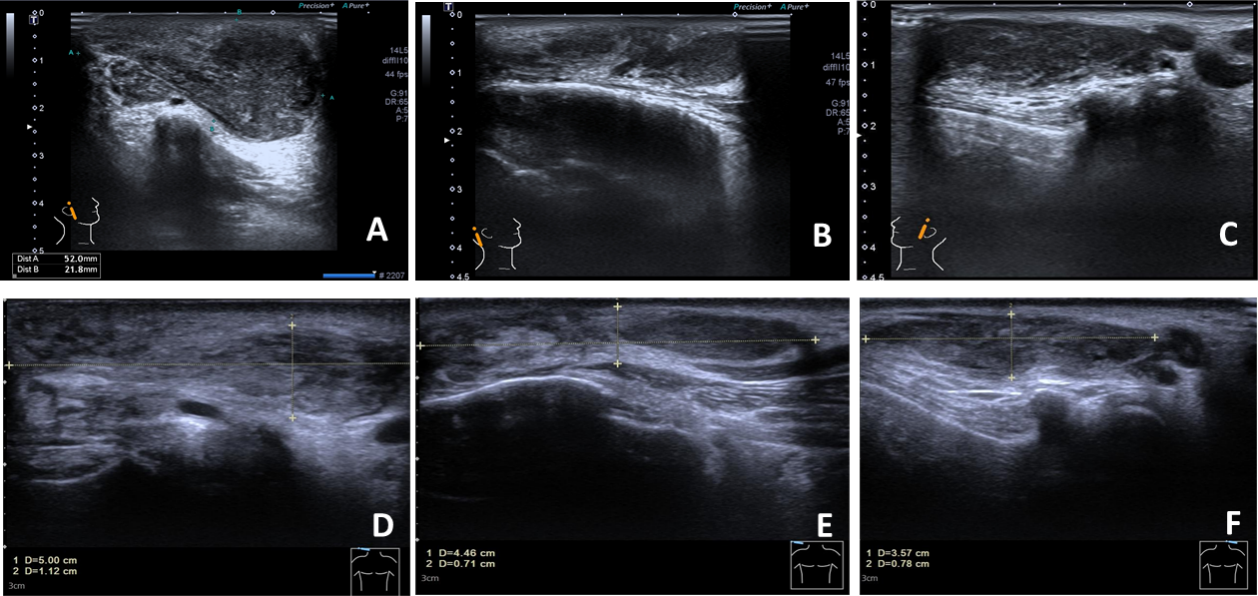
The anterior and posterior ears’ color Doppler ultrasound. The right anterior mass is in the size of 5.2×2.2 cm and has a heterogeneous hypoechoic–isoechoic appearance (right ear in front, right ear behind, and left ear in front); (A–C) before treatment; (D–F) after 16 weeks of treatment with dupilumab.
